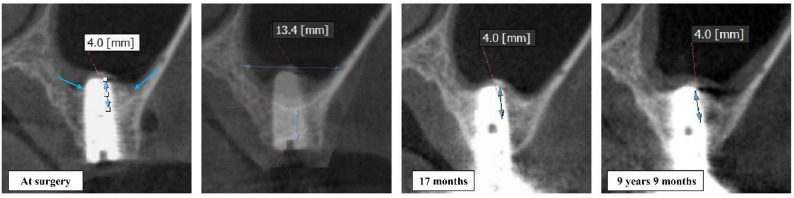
Method for measuring vertical bone gain. The vertical distance from the original sinus floor to the new bone–implant and the sinus width at the level of the implant apex was measured on CBCT cross-sections
